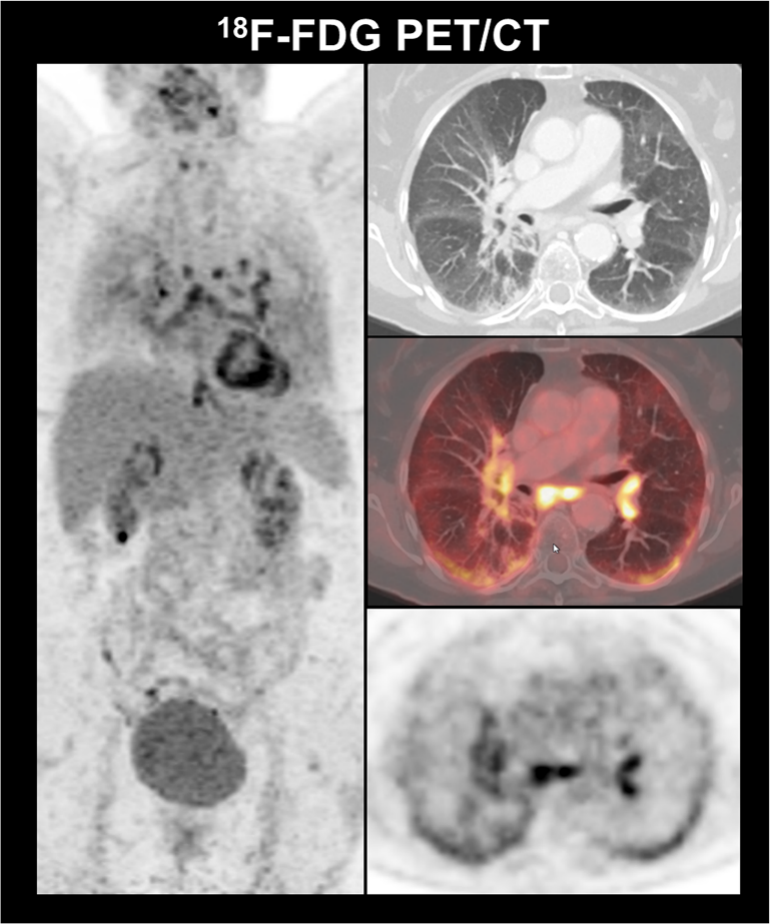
Identification of irAEs with FDG PET/CT in a 73-year-old patient with NSCLC cT3 N2 M0 (TNM 8th edition) who initially received concurrent chemoradiation with cisplatin/vinorelbine to a total dose of 63.6 Gy and was started on consolidation durvalumab. On follow-up FDG PET/CT, large ground glass opacities with consecutively elevated FDG-uptake were seen in both lungs. Moreover, newly enlarged and FDG-avid hilar lymph nodes could be observed. In sum, the findings were suggestive of immune-related pneumonitis and reactive lymphadenopathy. The patient was asymptomatic (grade 1) and as such no intervention was indicated.
